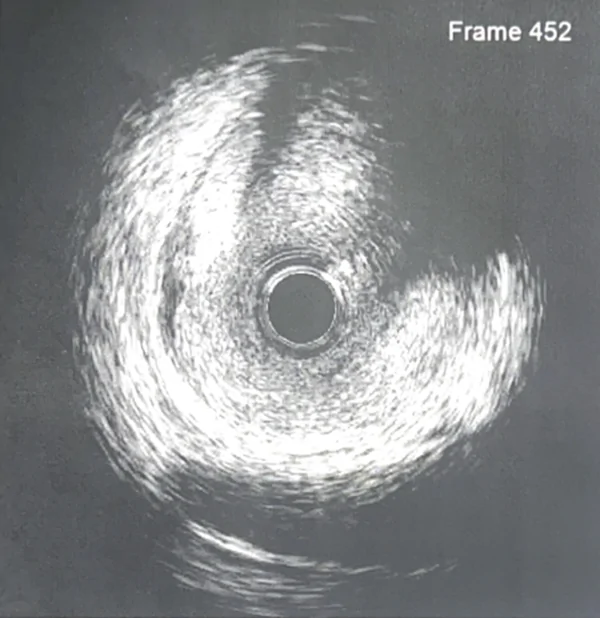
Poststenting intravascular ultrasound (IVUS) image of the left anterior descending artery. Following deployment of four overlapping drug‐eluting stents, IVUS demonstrates scaffolding and expansion of the true lumen within the treated segment.
